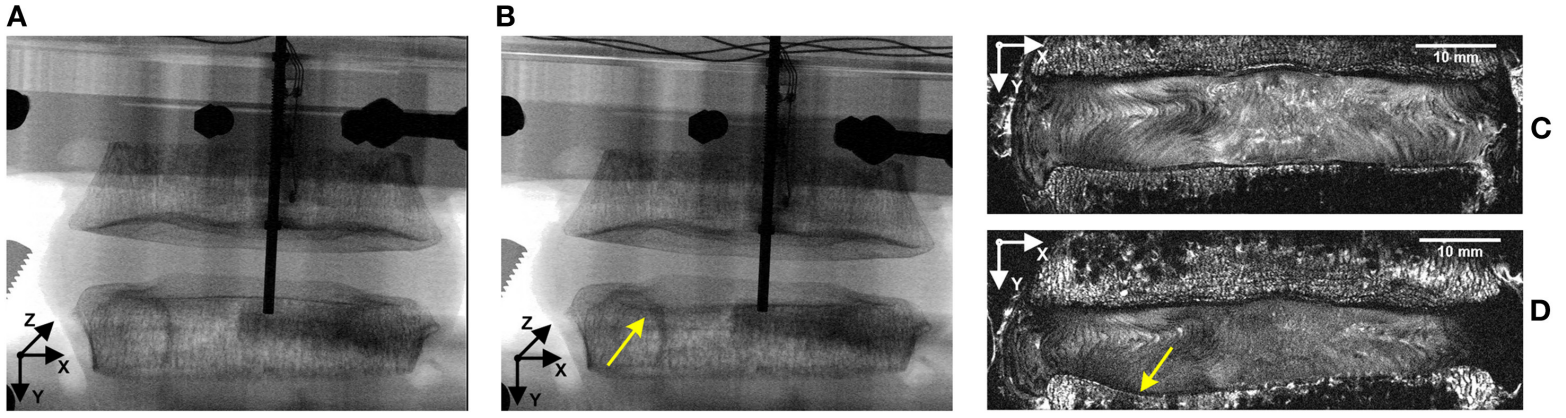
Representative fluoroscope (A,B) and MR (C,D) images of a sample before (A,C) and after (B,D) fracture. Combining these two imaging modalities, along with manual dissection of each sample allowed the location of fracture to be identified, with the fluoroscope images being used to identify the initiation of the fracture and the MR images being used to precisely locate the fracture site on a transverse slice. Yellow arrows identify the location of fracture.
